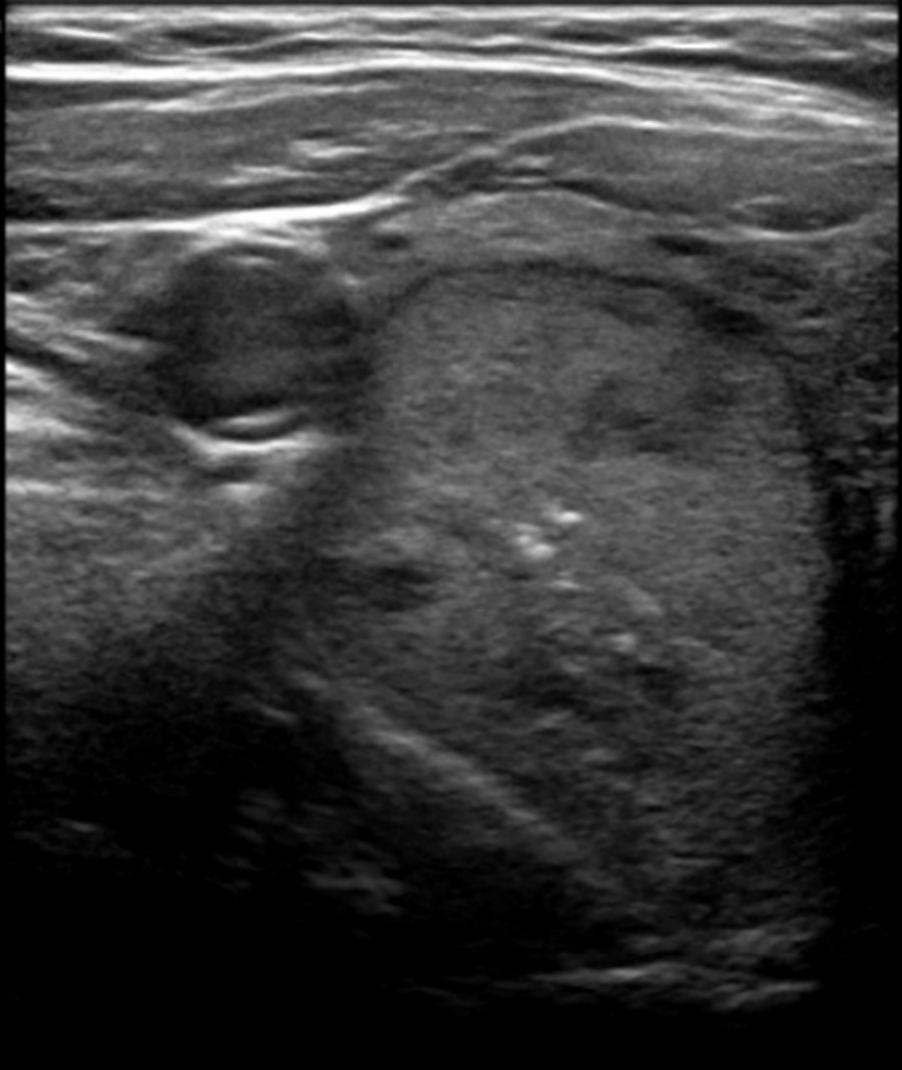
Axial ultrasound image showing a predominantly solid, isoechoic nodule in the right lobe with central punctate echogenic foci which was more difficult to classify using BTA. BTA, British Thyroid Association
